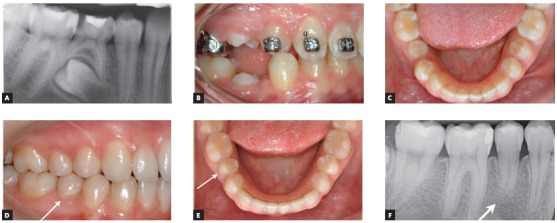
The ectopic position of the developing mandibular right second premolar was present on the panoramic radiograph of a 12-years old girl, who was seeking orthodontic treatment due to malposition of her maxillary teeth and Class II malocclusion (A). The premolar was severely distally tilted and the patient and her parents were interested to perform surgical uprighting (trans-alveolar transplantation) of the affected tooth instead of orthodontic extrusion to shorten the treatment time. The transplanted tooth erupted after few weeks into an oral cavity (B, C) and an orthodontic bracket was bonded after eruption into contacts with the opposing premolars. Normal occlusion was established after orthodontic treatment including the transplanted premolar (D, E; arrow). The root of the transplanted premolar continued development after surgery (arrow), but remained somehow shorter than normal (F). Pulp obliteration and normal hard periodontal tissues are also present on the radiograph.
